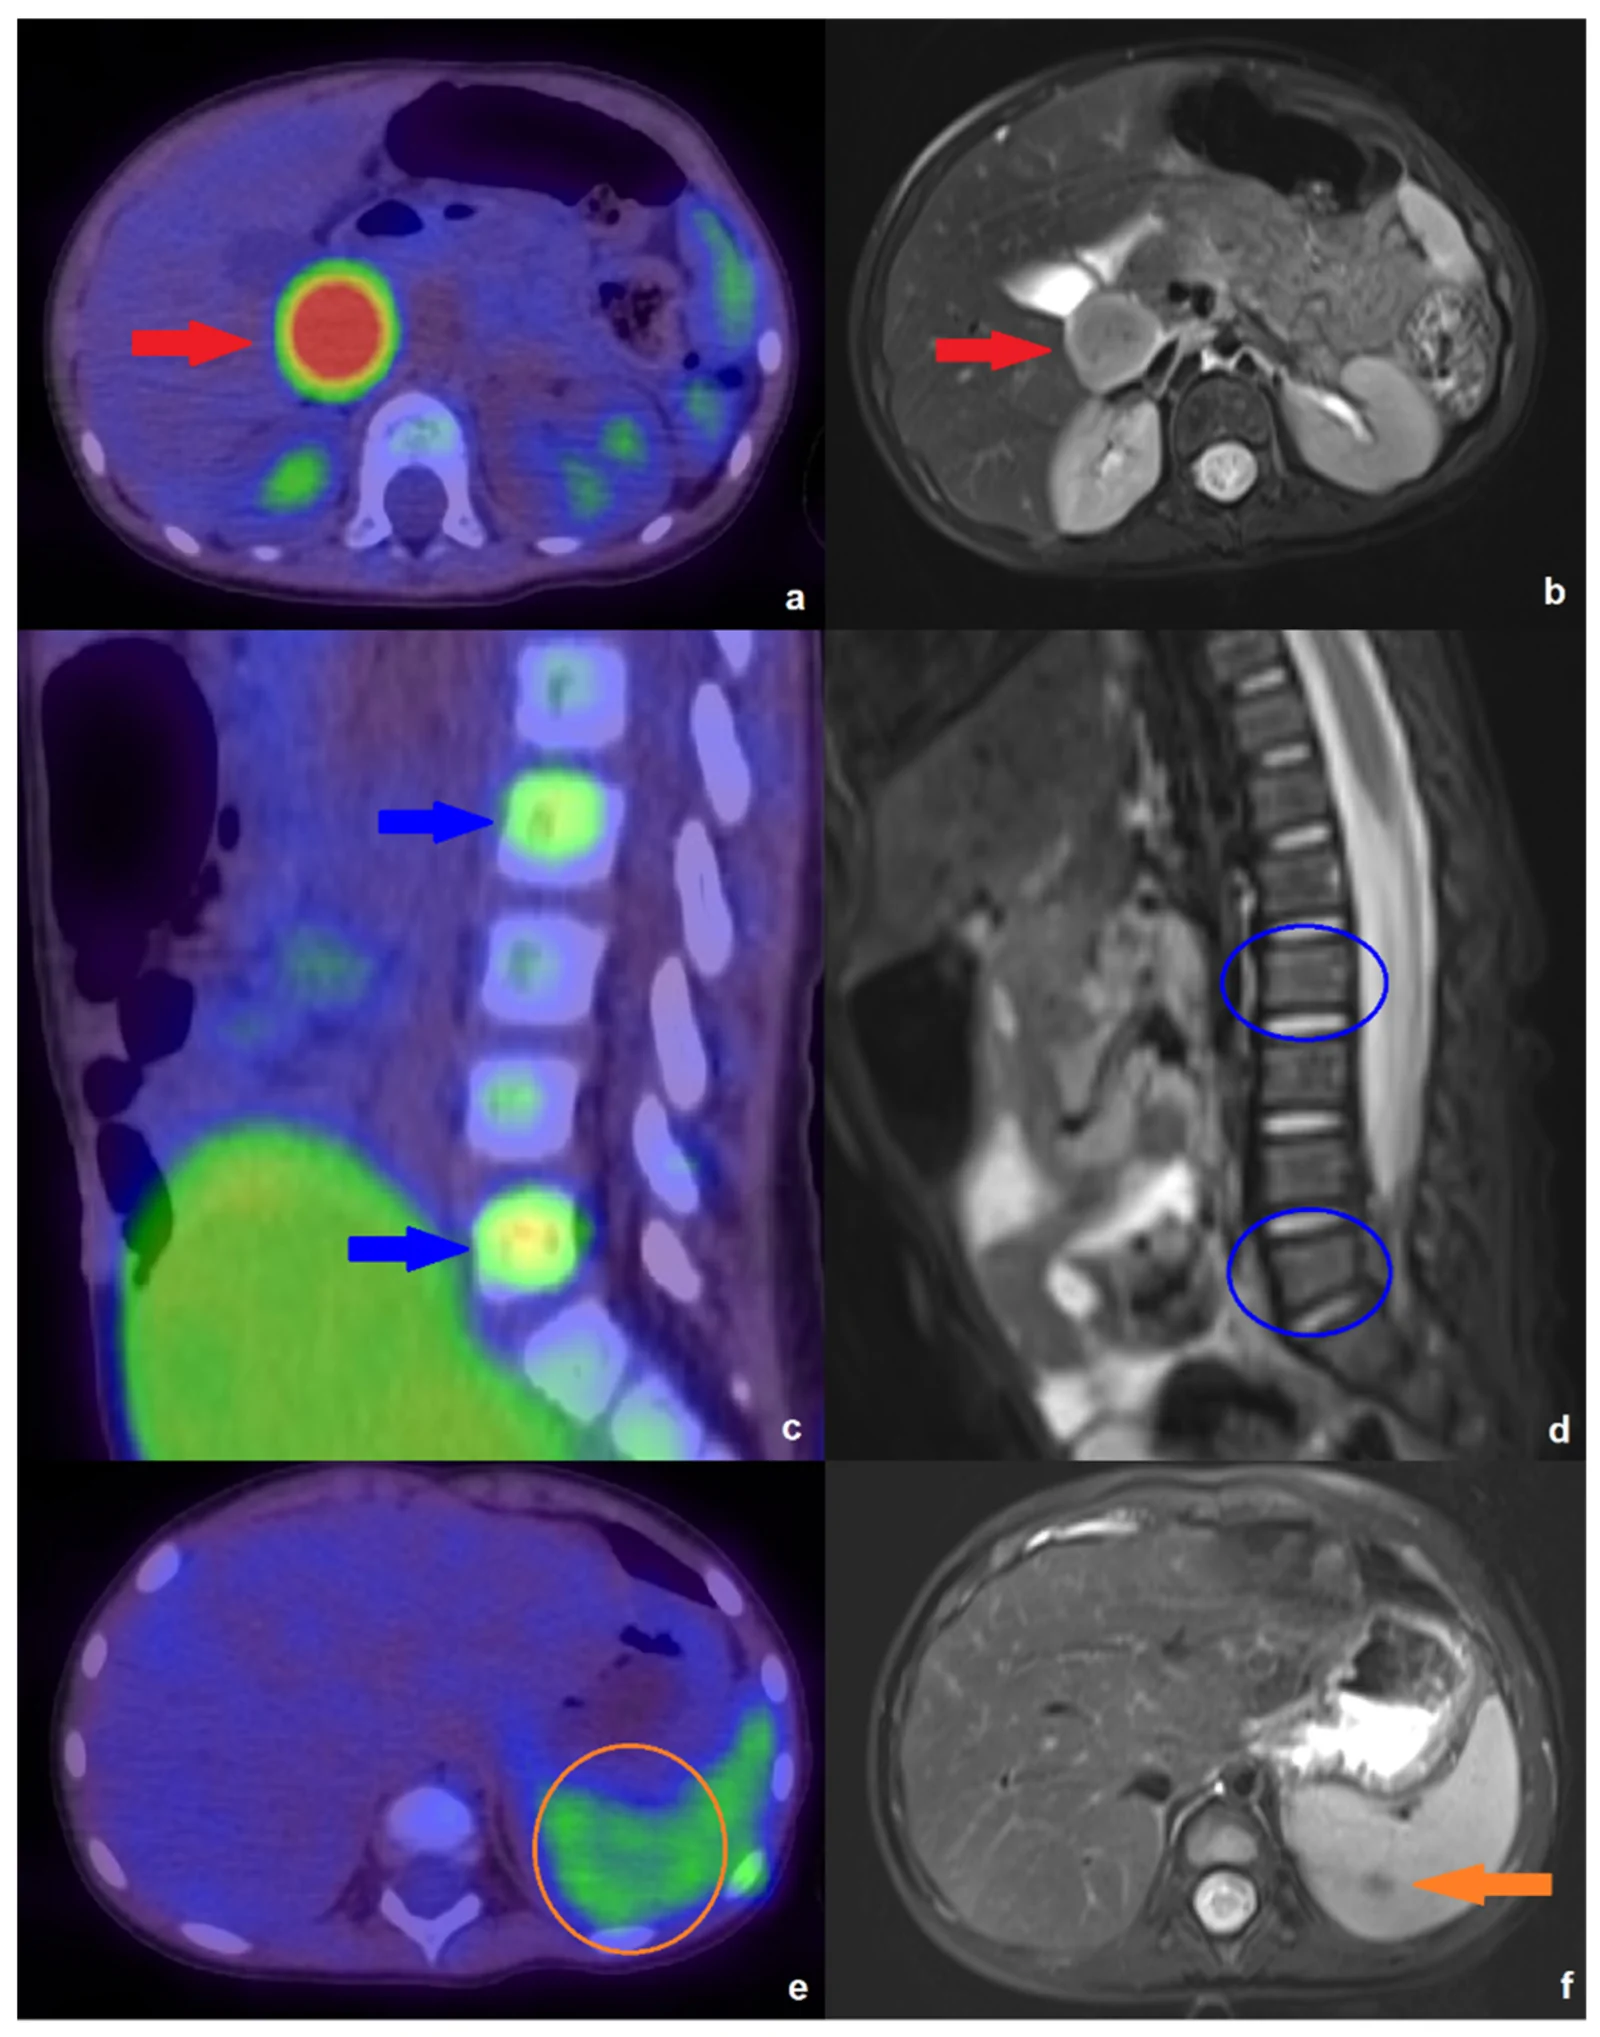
Fused PET/CT with FDG uptake being color-coded from low (blue) via green, yellow and orange to red (very high), (a) and MR (transverse T2-weighted TSE sequence with fat saturation), (b) images demonstrating an FDG-avid lesion in the right adrenal gland (red arrows). In the same patient, metastatic lesions in the lumbar spine were detected only by an increased FDG uptake (blue arrows, (c)) with no visible signal alterations in a corresponding TIRM MRI sequence (blue circles, (d)). In contrast, splenic involvement was not detected by PET/CT (orange circle, (e)), but was visible in the MRI (orange arrow, (f)).
